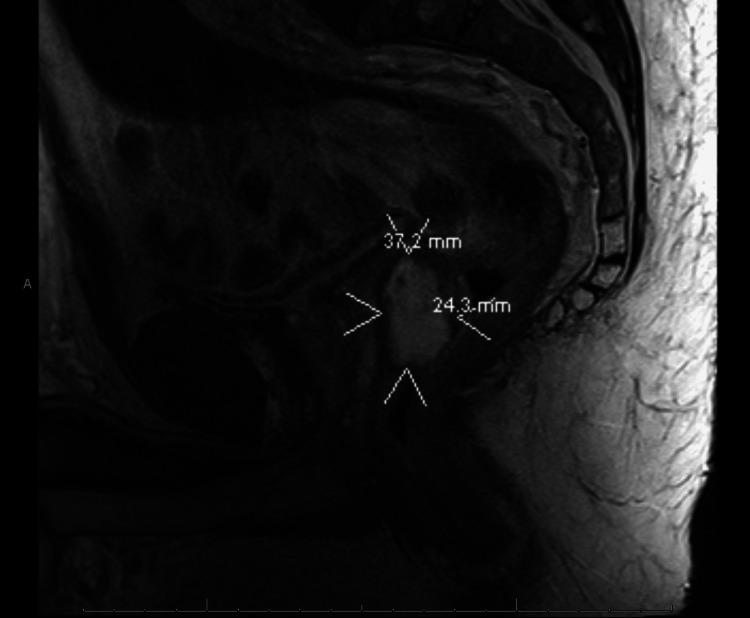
T2-weighted sagittal MRI of placement of SpaceOAR hydrogel in rectoprostatic space
